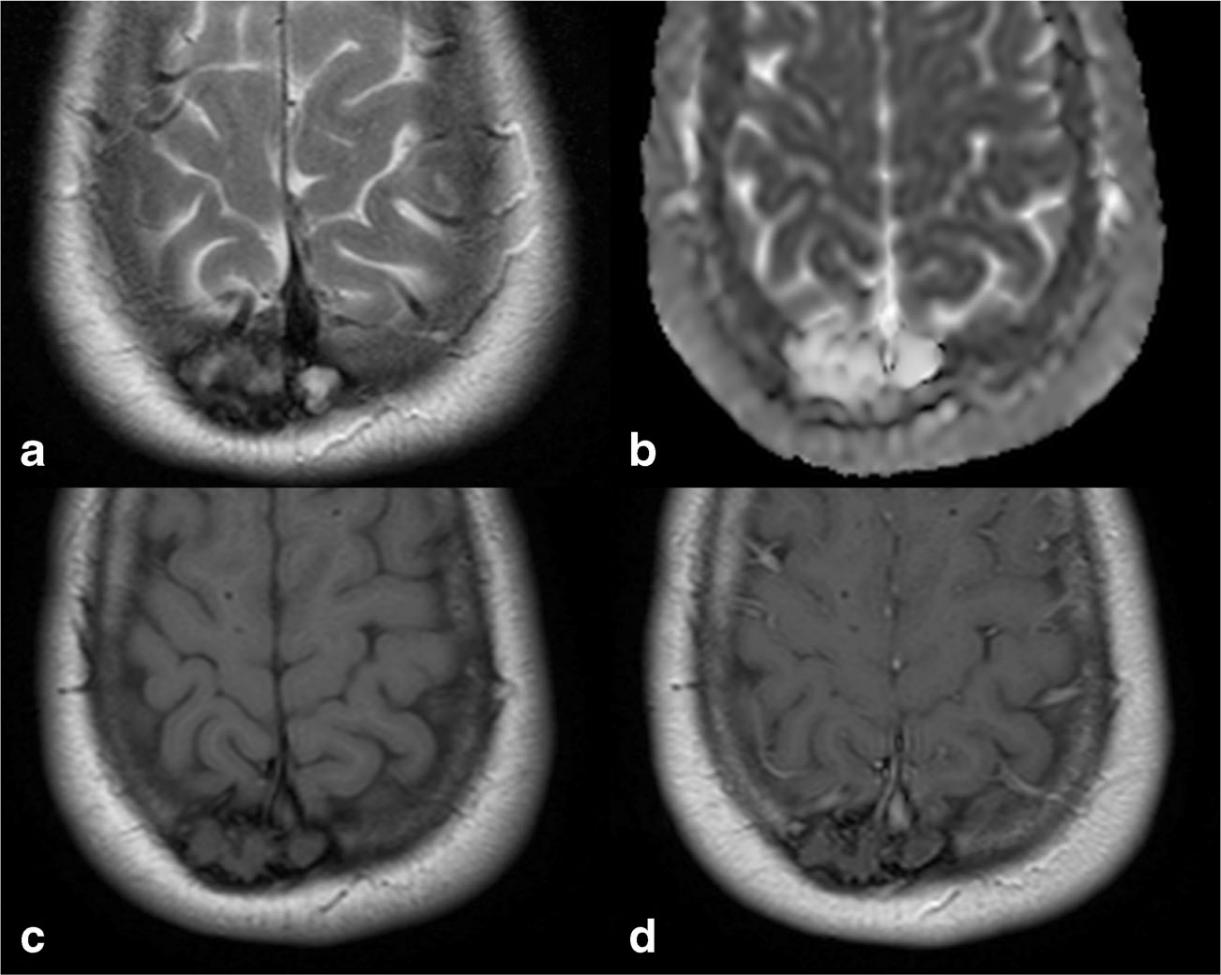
DF, same patient shown in Fig. 7. On MRI, the lesion presents a typical low signal intensity on T2w and T1w sequences (a, c), without diffusion restriction on the ADC map (b) or enhancement after contrast administration (d)
